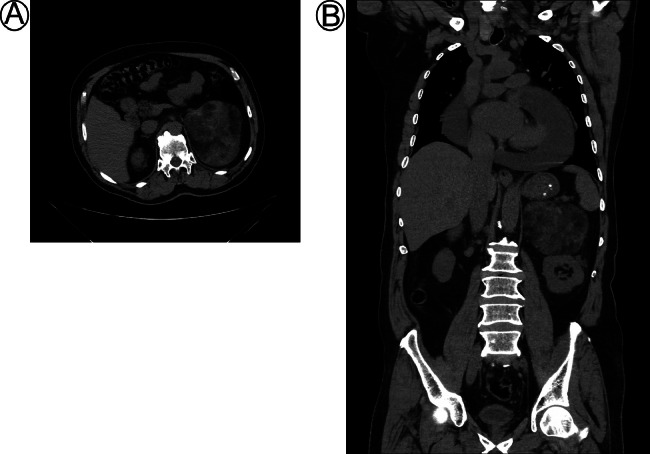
Abdominal Non contrast CT scan (A) axial section (B) coronal section revealed a well-defined lesion consisting of fat at left adrenal gland, the dimensions of the lesion were measured to be 12 × 10 × 8 cm in transverse x anteroposterior x craniocaudal dimensions, respectively.
